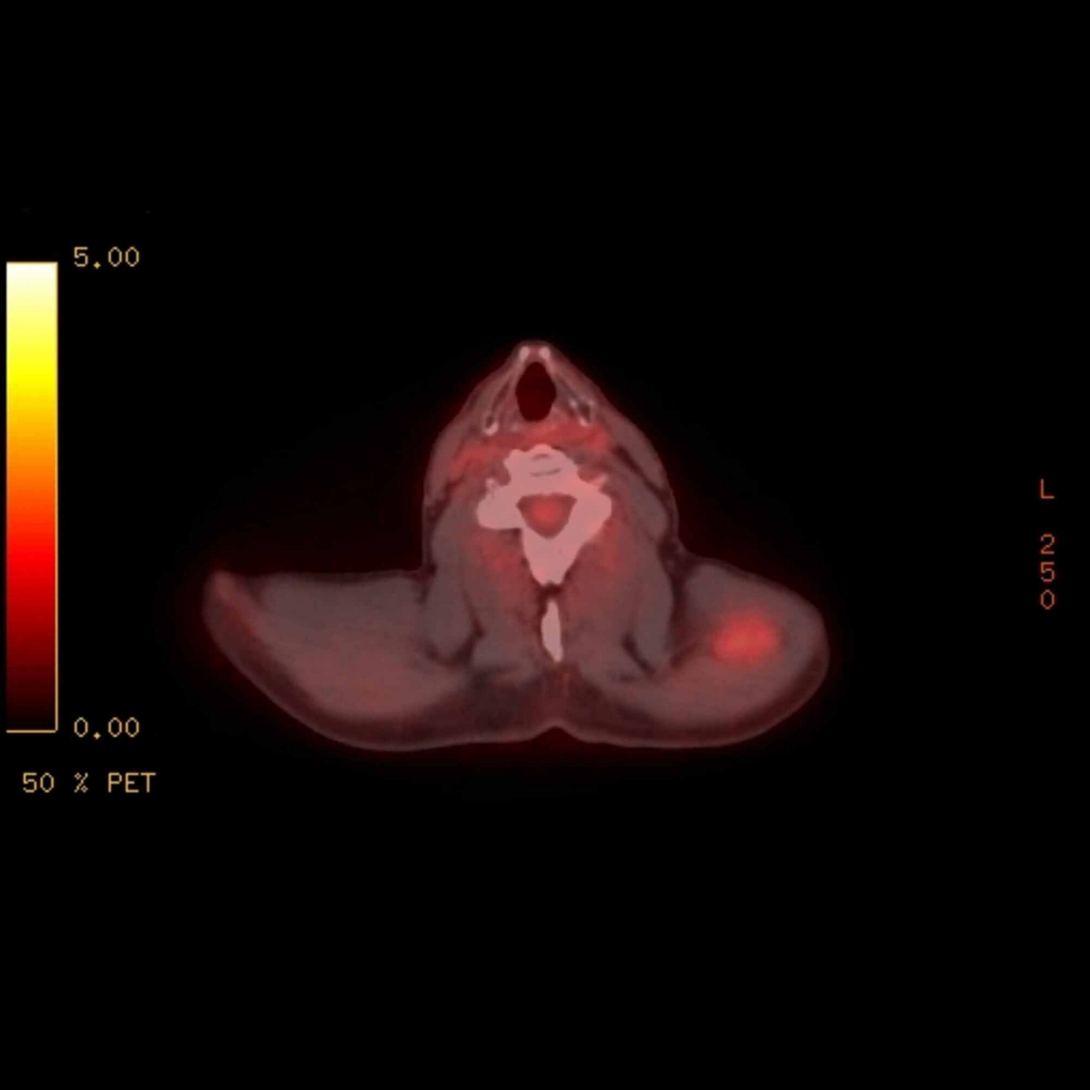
Positron-emission tomographic (PET)-CT scan showing increased uptake in the left trapezius muscle measuring 3.1 cm x 1.9 cm (standardized uptake values (SUV) 2.1).
